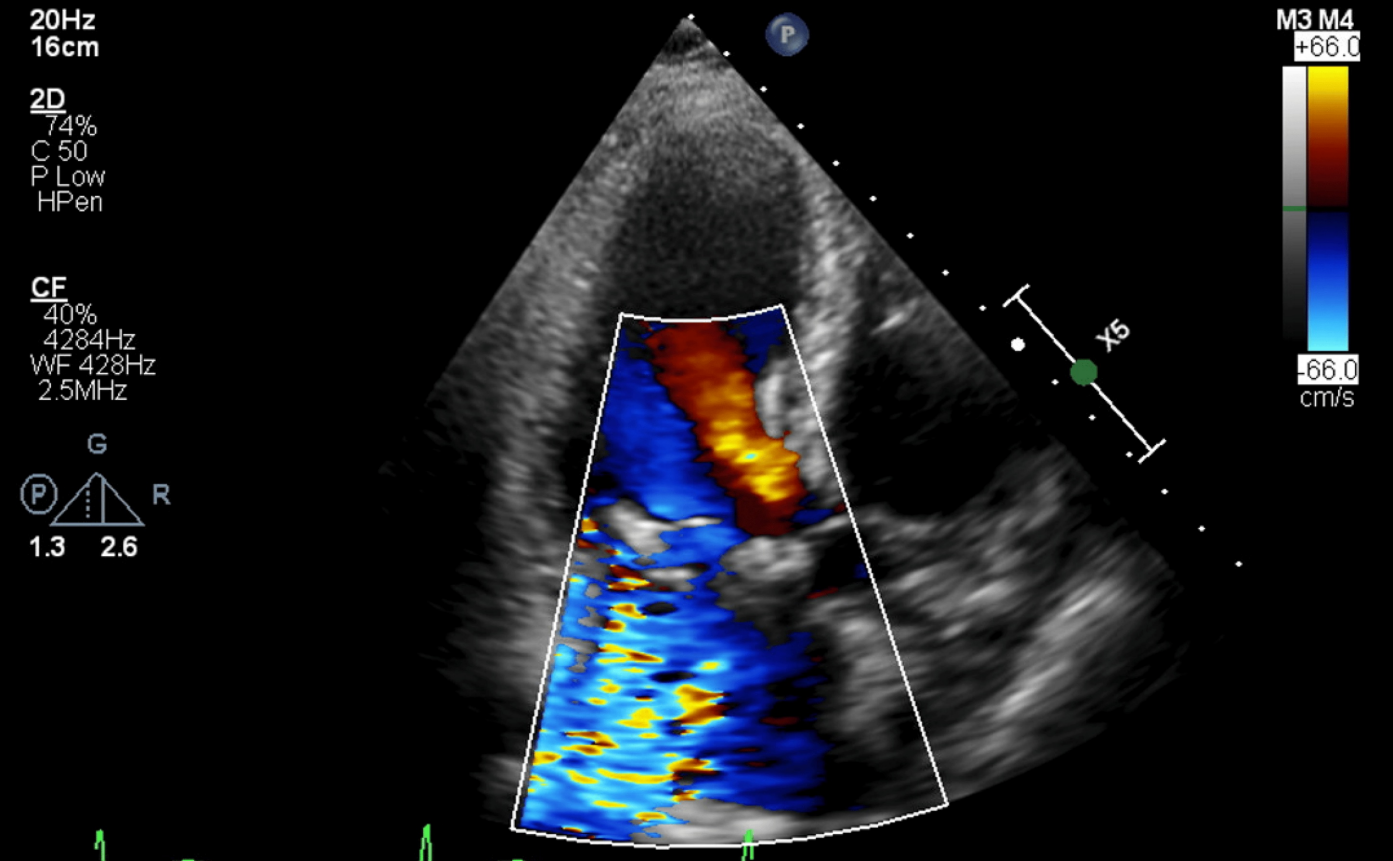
Transthoracic echocardiogram apical three-chamber view demonstrating paravalvular regurgitation.
